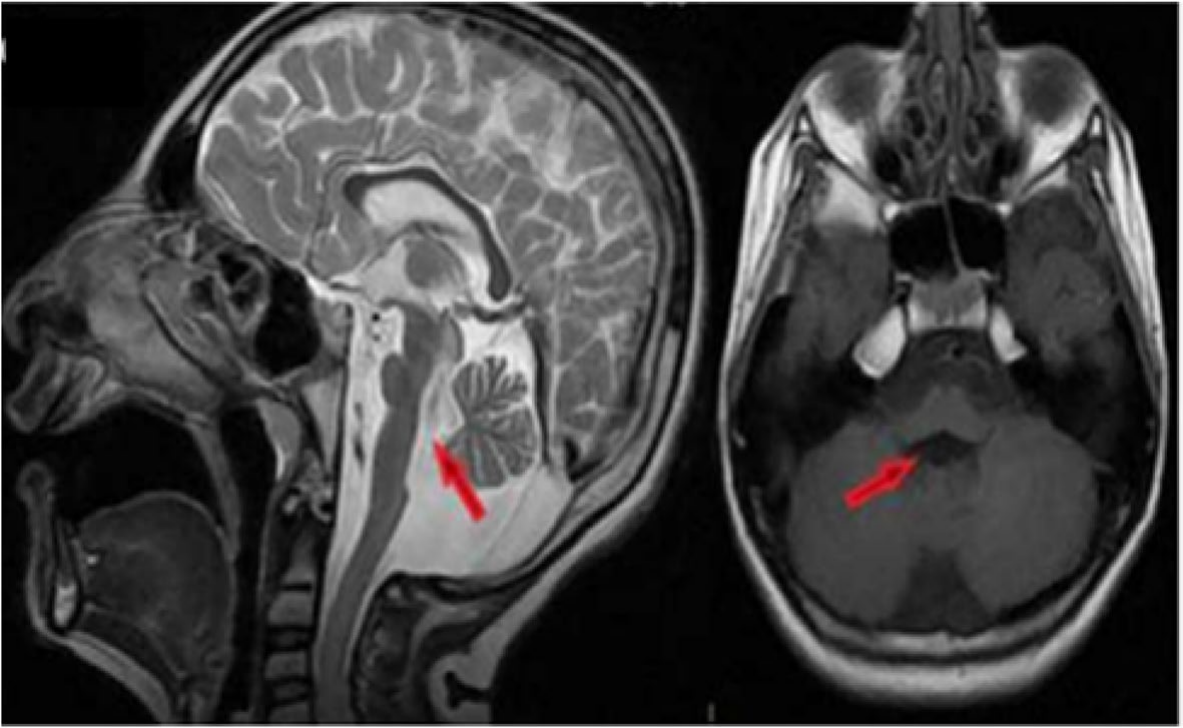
Six years old boy, brain MRI ( sagittal and axial sequences) is showing lateral enlargement of the ventricles and thinning of the corpus callosum and hypoplasia of the serebellum and pons (red arrow)
